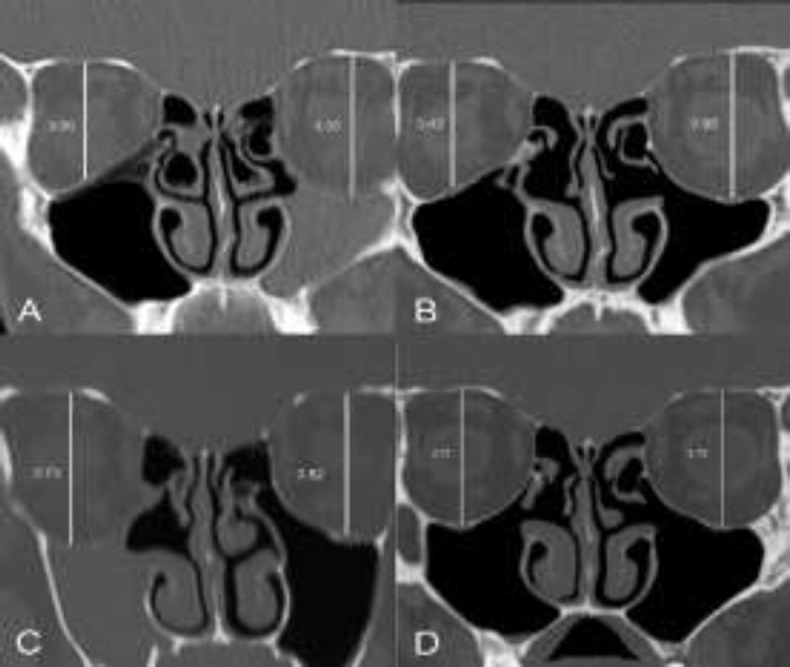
Coronal computed tomography scans showing the vertical dimension in centimeters of each orbit to demonstrate the grade of lowering of the maxillary sinus roof, A) Left silent sinus syndrome with uncinate process lateralization and maxillary sinus opacification before surgical operation. Evidence of a bilateral concha bullosa, B) Left maxillary sinus re-ventilated and bilateral concha bullosa correction. It is possible to notice a slight lateralization of the right uncinate process ten months after the first surgical operation, C) Evidence of right silent sinus syndrome with sinus opacification and lowering of the orbit two years after the first surgical operation, D) Post-operative computed tomography scan showing the re-ventilation of both maxillary sinuses 5 years after the second surgical operation. The orbital floors are at the same level on both sides
